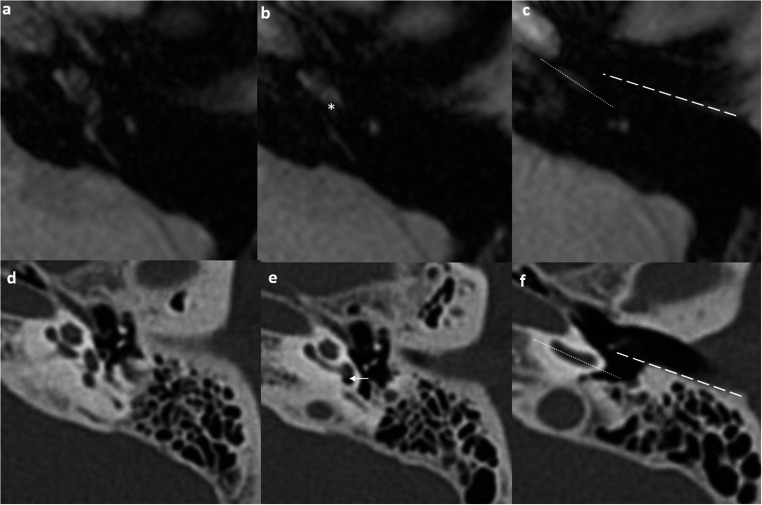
Standard axial MR section from the BB-MRI sequence where the measurements are performed (A to C). The axial section showing the basal turn of the cochlea in continuity to the vestibule is identified (A). The axial MR image one slice below the prior slice shows the dorsal aspect of the promontory of the basal turn of the cochlea (asterisk) as the surrogate for the round window niche for measurement of modified round window niche angle (B). The axial MR image two slices below the prior slice in b showing the mid external auditory canal (EAC) level for measurement of the EAC line (dotted line) and the basal turn line (dash line) (C). This slice allows the measurements of mastoid facial nerve location. Corresponding axial high-resolution CT showing similar axial sections as BB-MRI (D to F). The axial CT image showing the basal turn of the cochlea in continuity to the vestibule (D). The axial CT image showing round window niche (arrow) (E). The axial CT image at mid EAC level showing the EAC line (dotted line) and basal turn line (dash line) (F)
